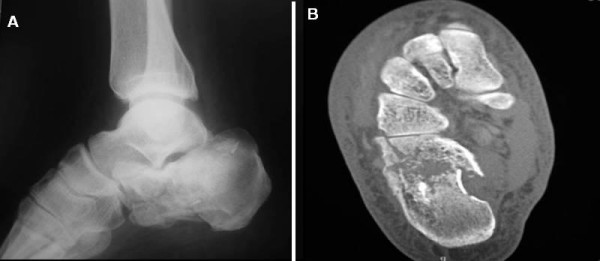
a) Preoperative lateral radiograph and (b) transverse plane CT scan demonstrating calcaneal fracture with intra-articular displacement.
